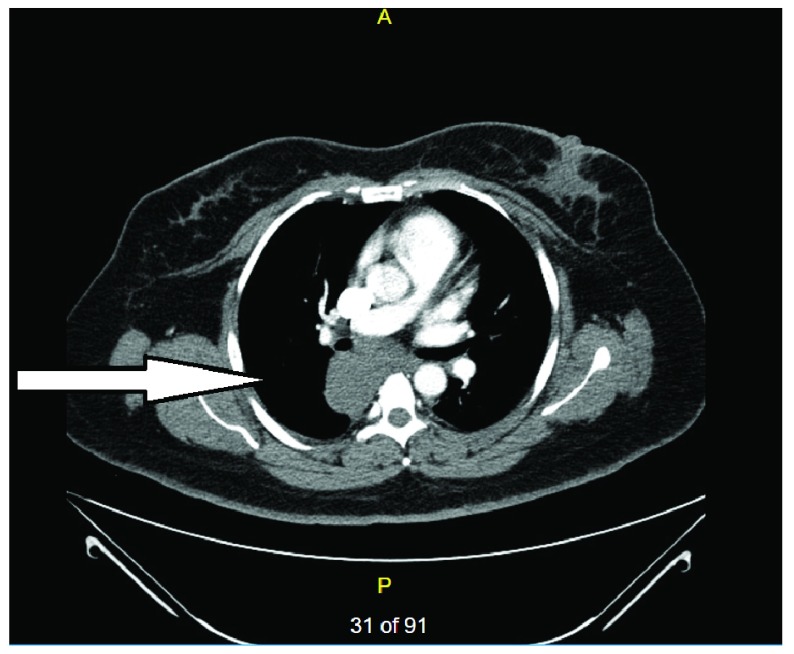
CT abdomen with contrast with chest window showing right subcarinal mass suggestive of right posterior mediastinal cyst measuring 5.4 X 5.0 cm (May 2015).
